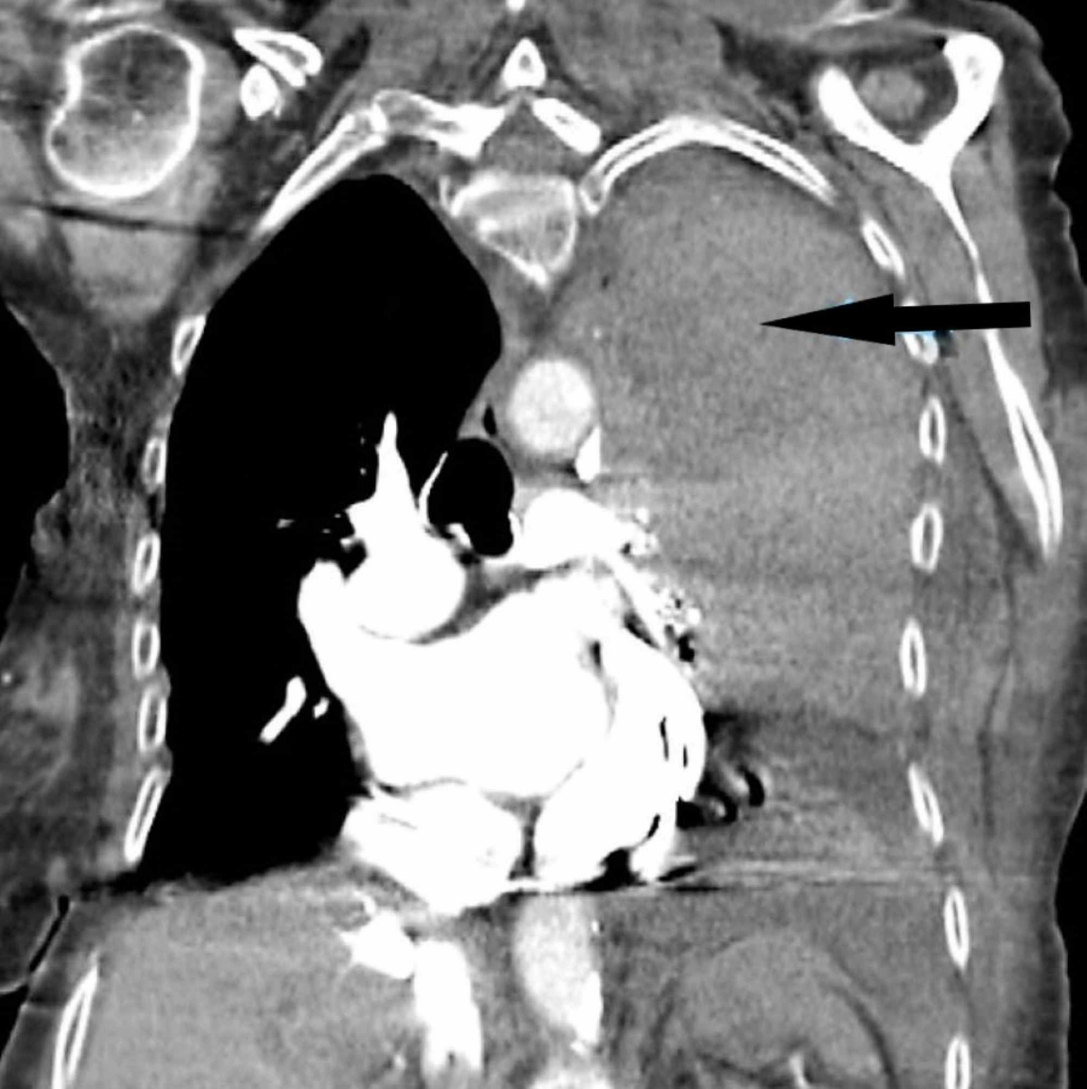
CT coronal plane image section post the placement of chest tube
